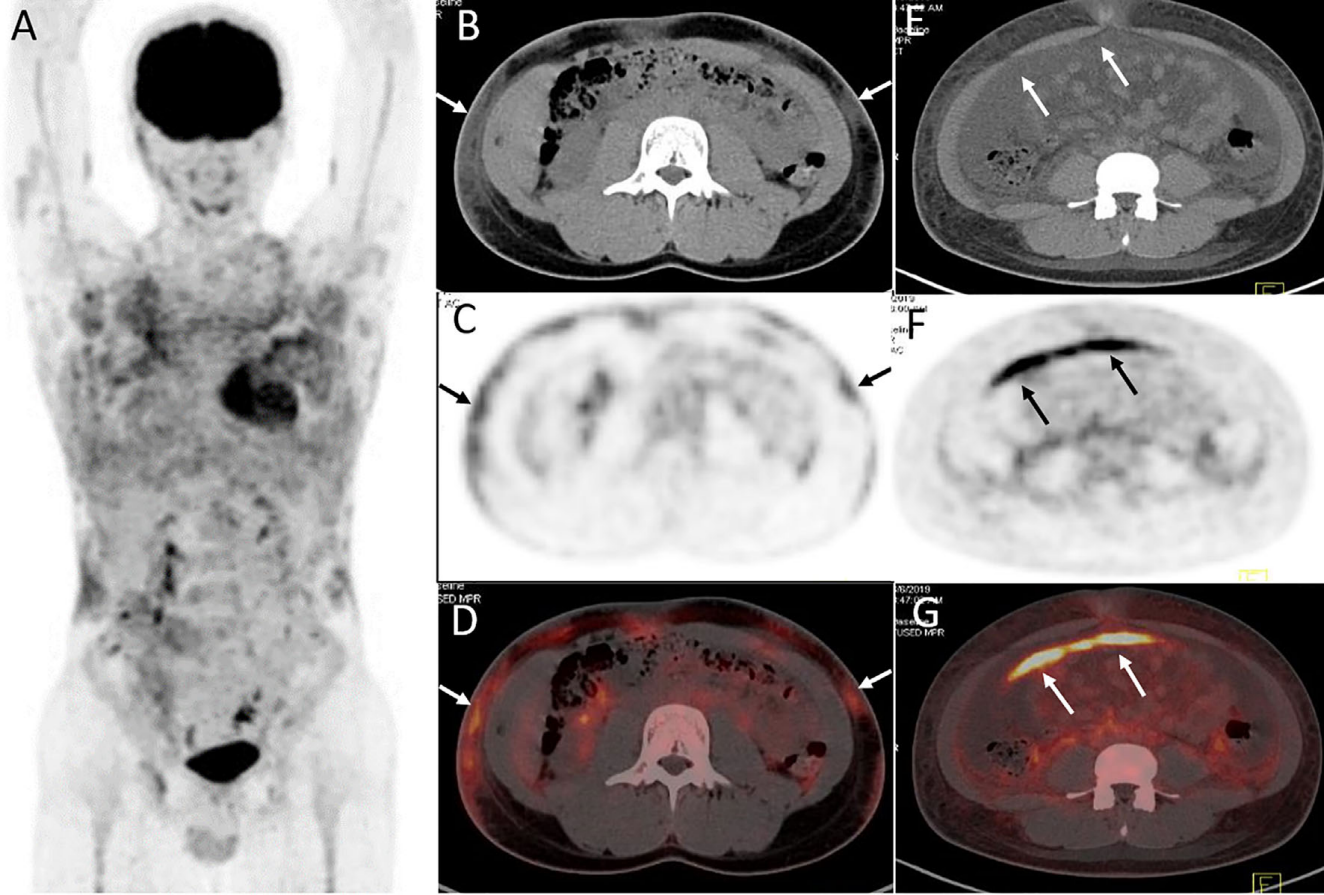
Twenty-year-old male with subcutaneous panniculitis-like T-cell lymphoma. Three-dimensional maximum intensity projection image (A), axial CT (B), corresponding PET (C) and fusion PET/CT (D) images from ^18^F-FDG PET/CT show hypermetabolic soft-tissue infiltrates subcutis diffusely in the trunk and both upper arms with ill-defined borders (arrows), which are the typical patterns of the disease. Abdominal axial CT (E), corresponding PET (F), and fusion PET/CT (G) images from ^18^F-FDG PET/CT clearly show a “strip-like” lesion with high uptake of ^18^F-FDG in mesentery, which represents lymphoma involvement but it is negative on CT scan.
